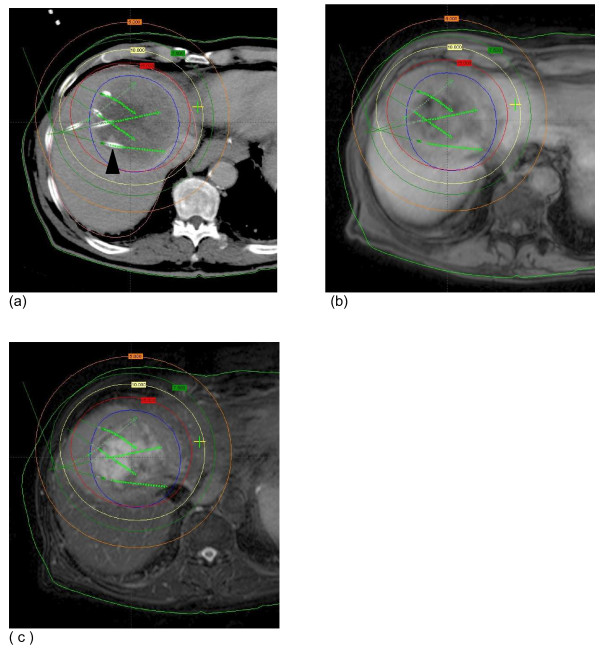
Image fusion of planning CT/dosimetry and follow-up MRI. (a) Contrast-enhanced CT after CT-guided positioning of 5 brachytherapy catheters in a colorectal metastasis (one catheter is labeled with a black arrowhead, the other catheter positions in cranial or caudal planes are indicated by green arrows). Isodoses lines the CTV (blue circle) after dosimetry with BrachyVision^® ^(b + c). MRI 3 months after brachytherapy: T1-w gradient echo 20 minutes post i.v. application of Gd-EOB DTPA (b) and T2-w UTSE FS (c) showing image fusion with the treatment planning CT.
